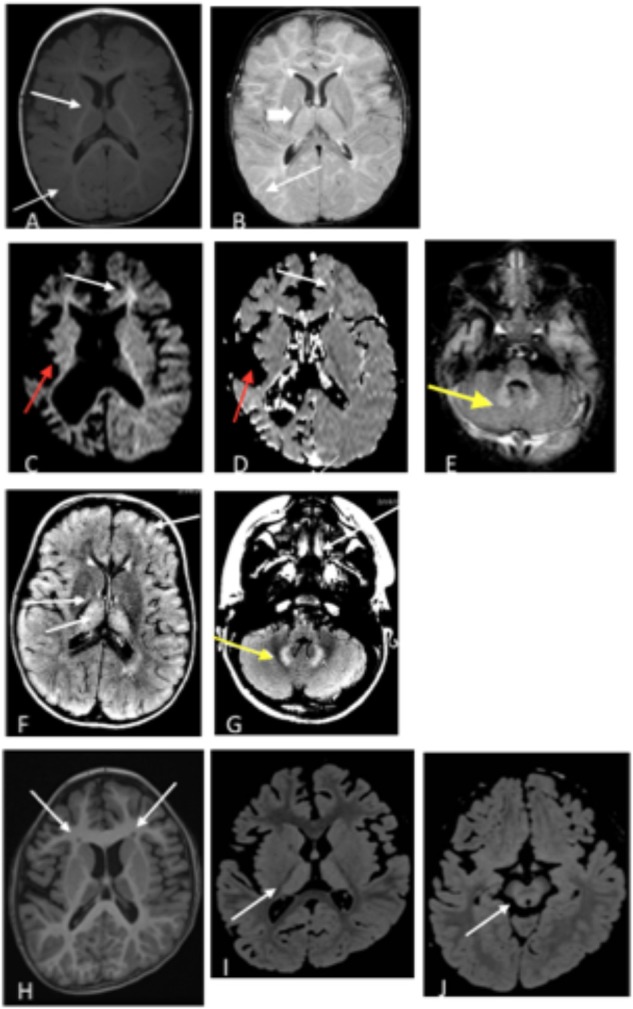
(A,B) Patient 1 at 9 months old. (A) T1 axial image and (B) T2 axial image. No evidence of atrophy or injury. White arrows demarcate areas of delayed myelination; thick arrow show abnormal hyperintensity in bilateral thalamus. Images (C–E). Patient 2 at 17 months old. (C) Axial diffusion weighted imaging (DWI) and (D) Axial apparent diffusion coefficient (ADC). Severe bilateral cerebral atrophy right side (red arrows) greater than the left side. Enlarged lateral ventricles secondary to ex vacuo loss. White arrows demonstrate acute restricted diffusion in white matter than can be related to acute injury. (E) Axial T2 FLAIR image with T2 hyperintensities indicated by yellow arrow in bilateral dentate nuclei. (F,G) Patient 3, age 3 years. T2 FLAIR axial images are shown. (F) White arrow shows T2 hyperintensities in bilateral thalami, and (G) Yellow arrow shows T2 hyperintensities in dentate nucleus. (H–J) Patient 4 at age 6 years. (H) Axial T1 image shows bilateral frontal periventricular heterotopia (white arrows). (I,J) Axial FLAIR images showed patchy hyperintensity in the thalamus (I) and midbrain (J) (white arrows).
